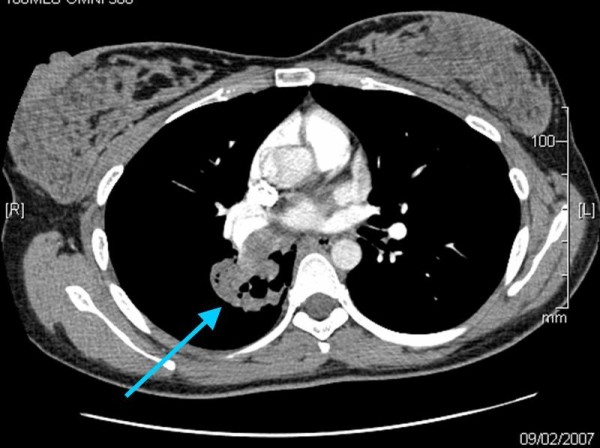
Computed tomography pulmonary angiogram demonstrating cavitating mass in right lung.
